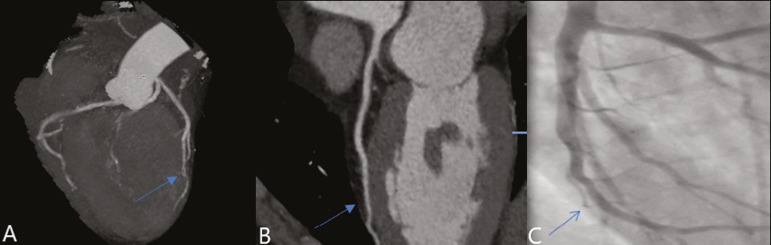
Myocardial bridge in the ADA. A: Maximum intensity projection reconstruction. B: CTA. C: Catheterization.
